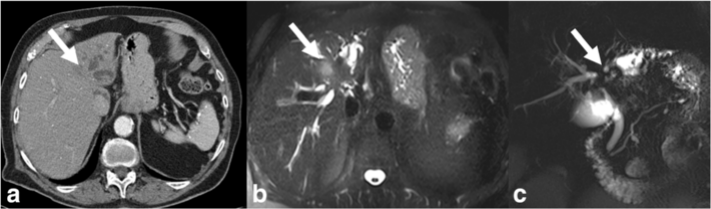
Peripheral cholangiocarcinoma with involvement of confluence. Infiltrating pCCA of the left hepatic duct (arrow) isodense to liver parenchyma on axial contrast enhanced CT (a) with dilation of the left hepatic ducts. The ductal thickening is hyperintense (arrow) on T2-weighted MRI image (b) with extension to the confluence causing mild dilatation of the right hepatic ducts demonstrated better on MRCP (c).
